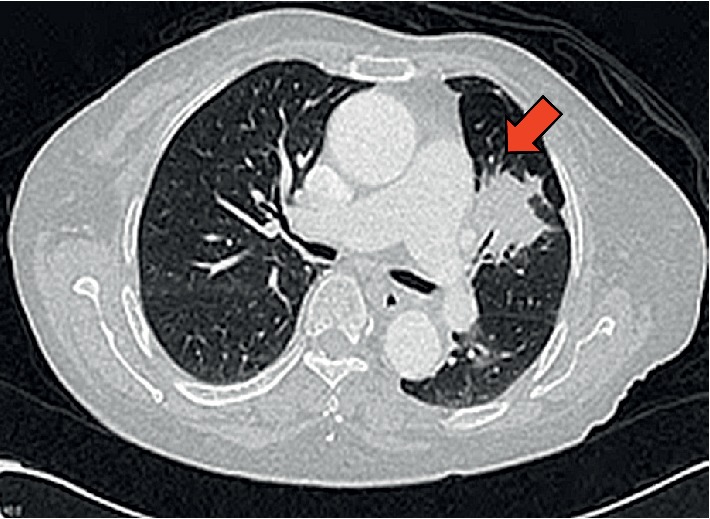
CT thorax showed a left upper lobe neoplastic lesion (red arrow) with multiple lymphadenopathies in the mediastinum.
